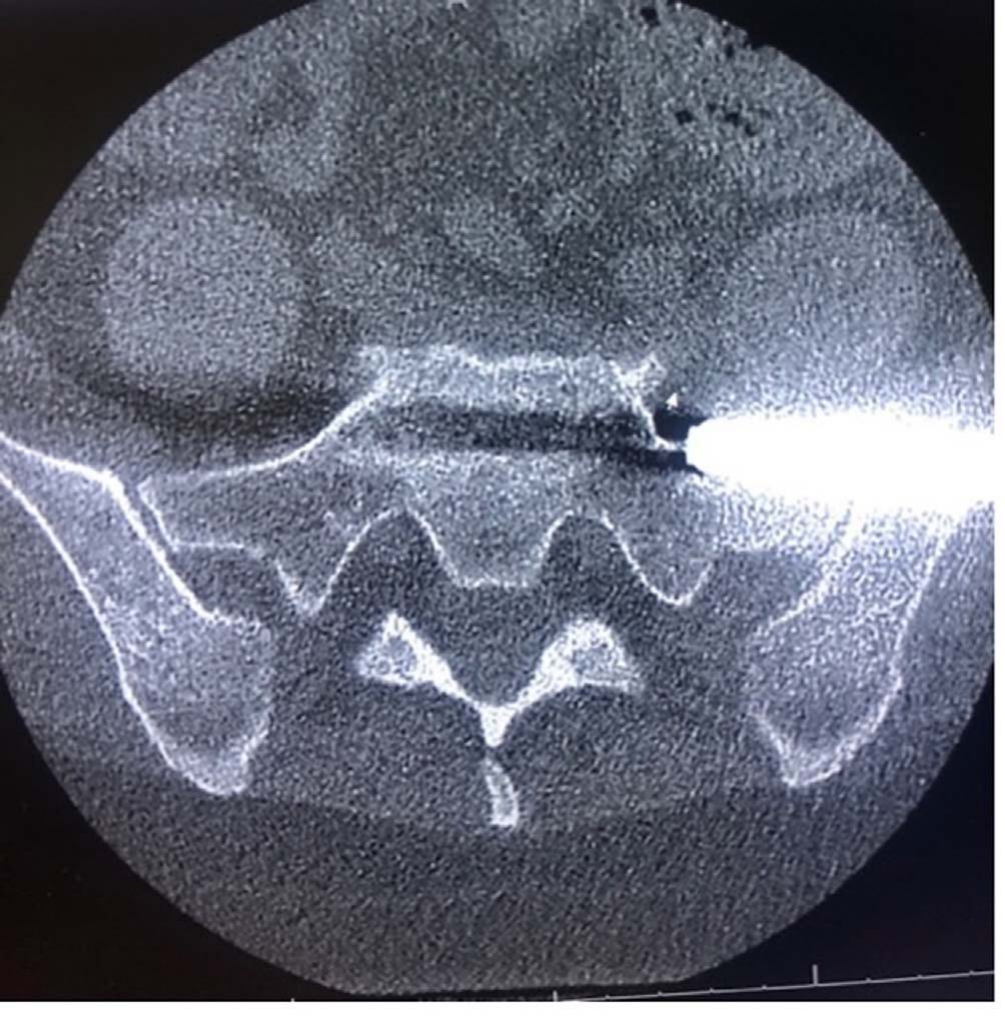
Axial cuts of a CT scan showing sacrum and both sacroiliac joints, note the implant breaches the left sacroiliac corridor anteriorly.
